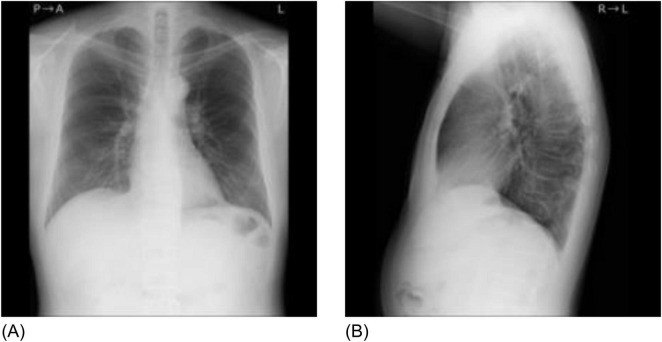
Sample chest radiographs of one patient used in this study. (A) Frontal chest radiograph, (B) Lateral chest radiograph. The original images were down-sampled and zero-padded to 1,024 × 1,024 matrixes with the aspect ratio preserved and resized to 299 × 299 as the input size for the pre-trained model.
